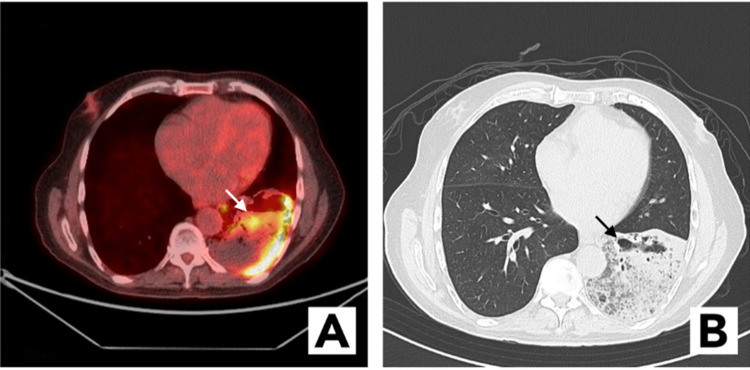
(A) Positron emission tomography-computed tomography image with fluorodeoxyglucose-avid areas in the left lower lobe. (B) Computed tomography image with large consolidative changes and ground-glass opacities in the left lower lobe.
